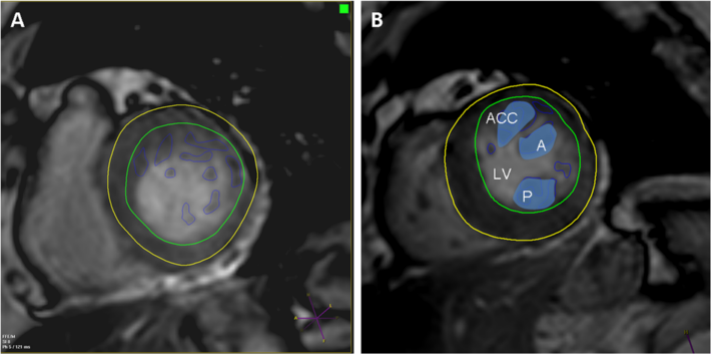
Cardiac MRI, short axis view. (A) Unremarkable LV wall abnormality with normal LV mass at end-diastolic phase by MRI; (B) Hypertrophy PMs as well as existence of accessory PM (ACC) shown.
